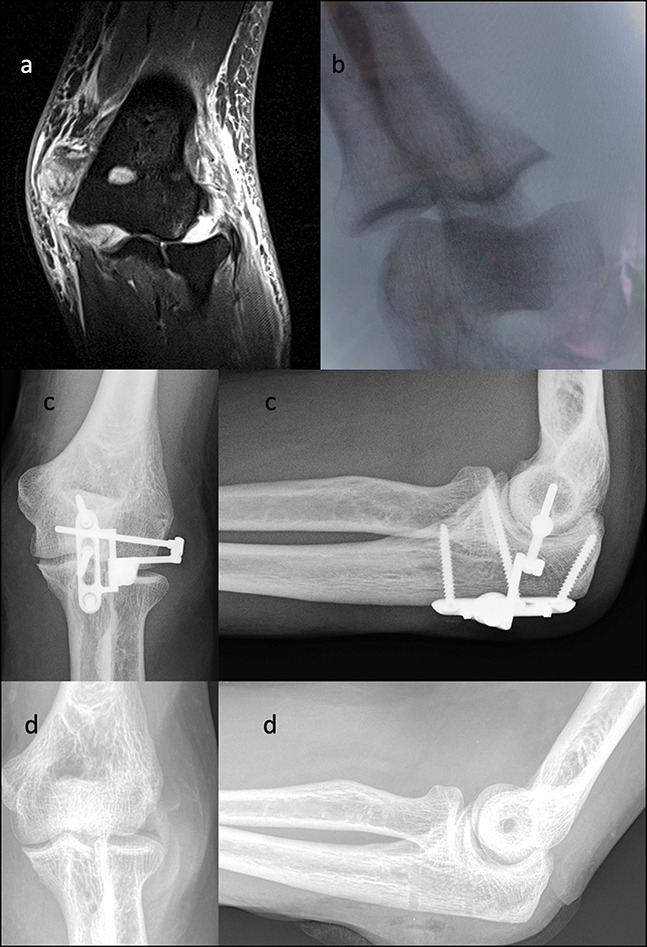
Case 1: Magnetic resonance image (A) and fluoroscopy image (B) demonstrating gross instability of the elbow with medial and lateral joint widening. Radiographs demonstrating surgical treatment using an internal joint stabilizer to provide stability and maintain a concentric joint (C). Twelve-month follow-up radiographs showing a reduced and stable joint (D).
